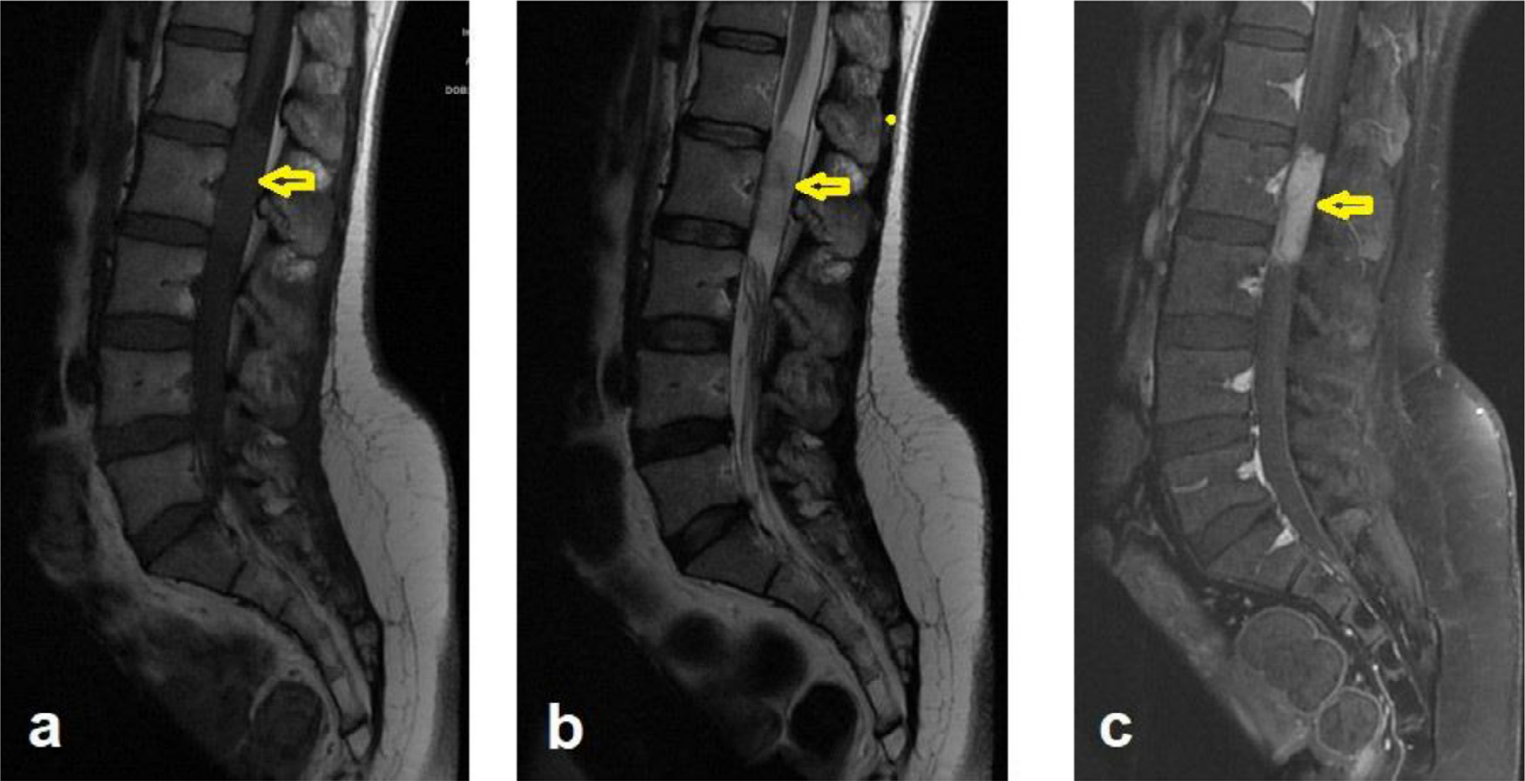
Sagittal MRI of the lumbar spine demonstrates an extramedullary, intradural spinal mass centered at L2 level. The lesion is low/isointense on T1 (A), heterogenous signal on T2 (B), and homogenously enhancing after contrast administration (C)
